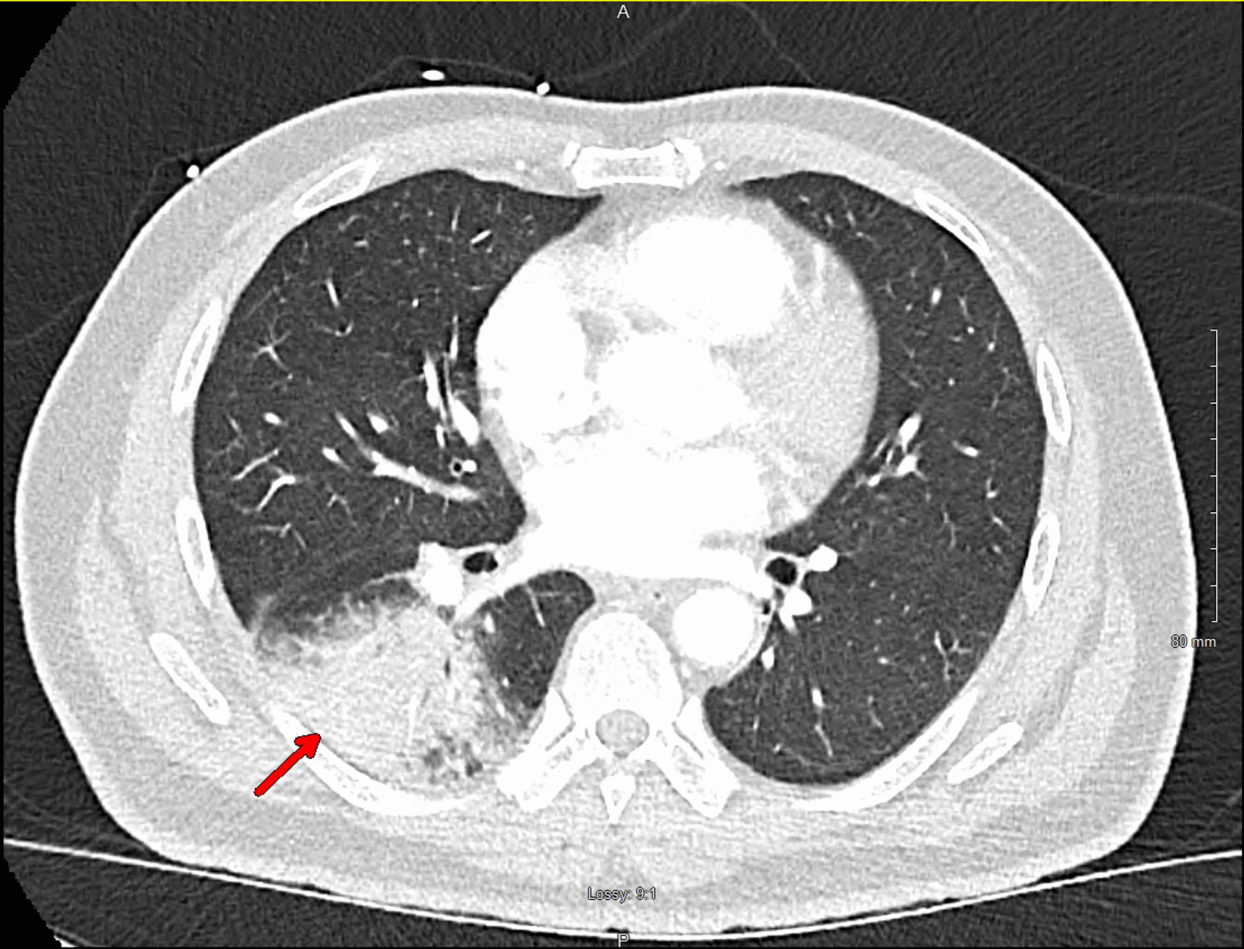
CT scan of the chest showing right lower lobe consolidation.
